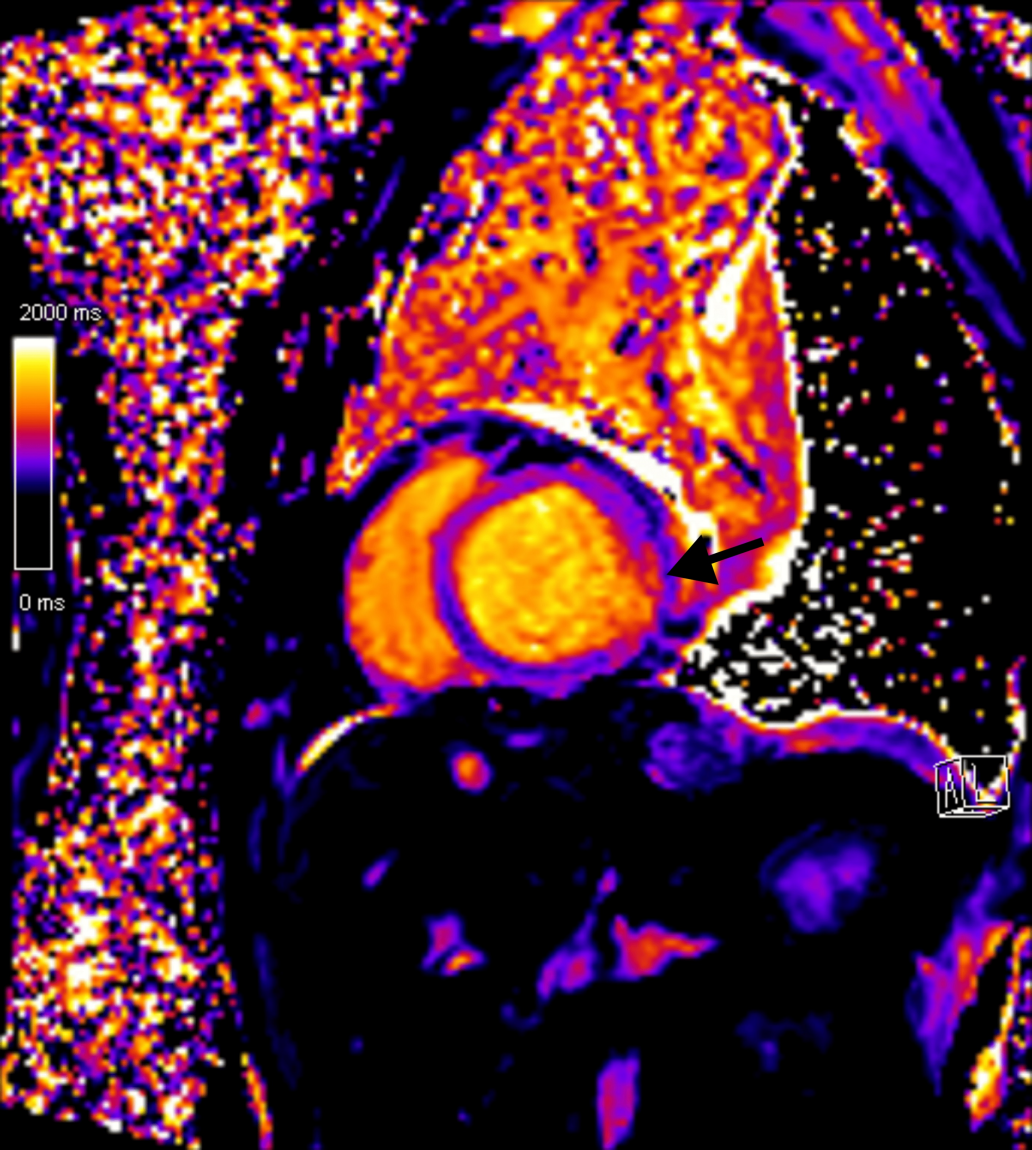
Native T1 mapping cardiac MRI sequence Increased native T1 mapping values in the inferolateral segment. MRI, magnetic resonance imaging.
